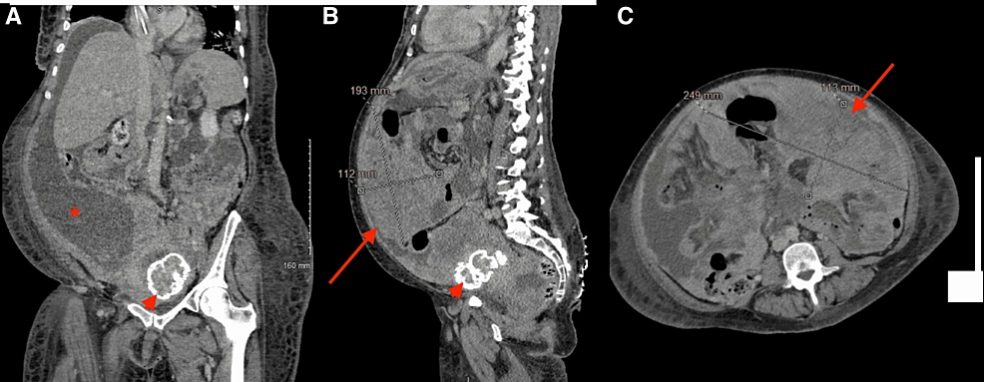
CT scan of the abdomen and pelvis. (A) A coronal section showing ascites (asterisk), the liver is seen without metastatic disease, normal size, and contour. (B) A sagittal section shows a large peritoneal mass (arrow) of 19 x 25 x 11 cm, also seen on (C) the axial section. Arrowhead points toward an incidental calcified fibroid seen in (A) and (B).
